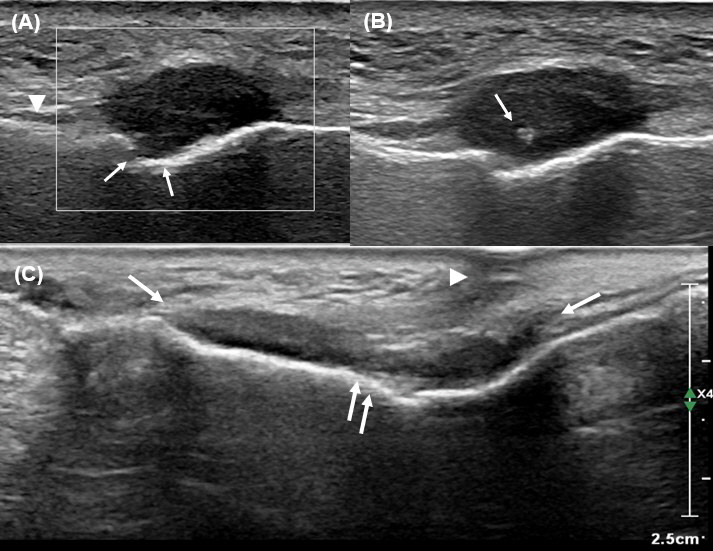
Face ultrasonography (US). (A) Longitudinal US image shows a focal hypoechoic nodular lesion in the right premaxillary fat pad, involving the levator labii superioris muscle (arrowhead) and abutting the infraorbital nerve branch (arrows). No internal vascularity is seen on Doppler, suggestive of a non-vascular soft tissue lesion. (B) US-guided fine needle aspiration biopsy was performed during the same session (needle tip: arrow), targeting the hypoechoic component. (C) On follow-up US performed 5 months later, the lesion shows interval growth in both size and extent (arrows), with newly developed overlying skin retraction and hypoechoic changes in the subcutaneous fat layer (arrowhead), suggesting progression with superficial extension.
